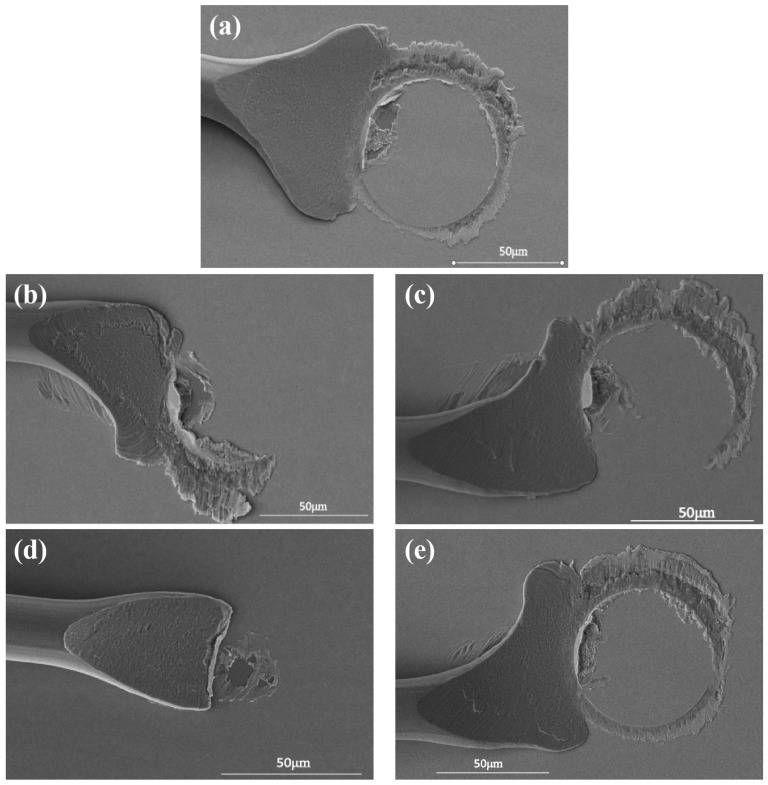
Morphology of Pd80 wedge-bonded points under different bonding pressure/ultrasonic power ((a) 45 g/105 mW; (b) 45 g/95 mW; (c) 45 g/115 mW; (d) 40 g/105 mW; (e) 50 g/105 mW).
